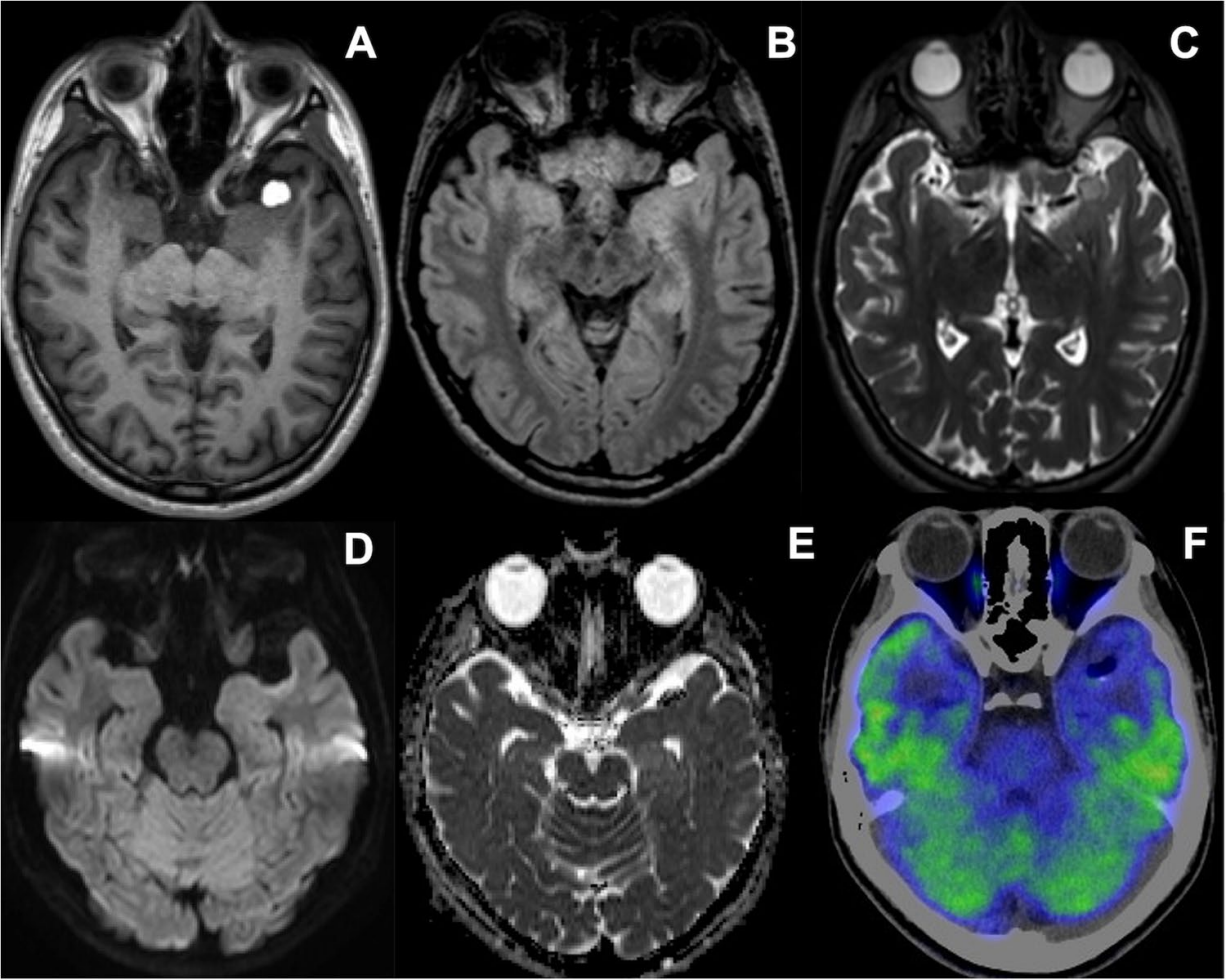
The Sylvian lipoma shows a markedly high signal intensity on axial T1-weighted imaging (A) and fluid-attenuated inversion recovery imaging (B), along with a slightly high signal intensity on axial T2-weighted imaging (C) on preoperative magnetic resonance imaging. The Sylvian lipoma shows signal supression on axial diffusion-weighted imaging (D) and the corresponding apparent diffusion coefficient map (E). The anterior temporal cortex adjacent to the Sylvian lipoma shows hypometabolism on fluorodeoxyglucose positron emission tomography/computed tomography (F)
